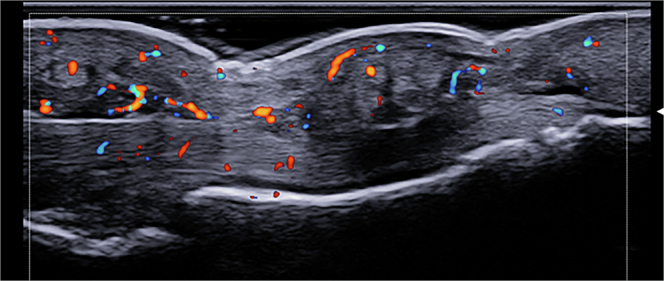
Ultrasound features of active dactylitis. Gray-scale longitudinal reconstruction of the volar aspect of a finger affected by active dactylitis, obtained through multiple scans, showing thickened, iso-hypoechoic subcutaneous edema and distension of the flexor tendon sheath surrounding the tendon. Power Doppler imaging demonstrates a scalloped appearance of the flexor tendon in regions lacking pulley support, with marked intra-tendinous hypervascularisation. (PD power Doppler).
